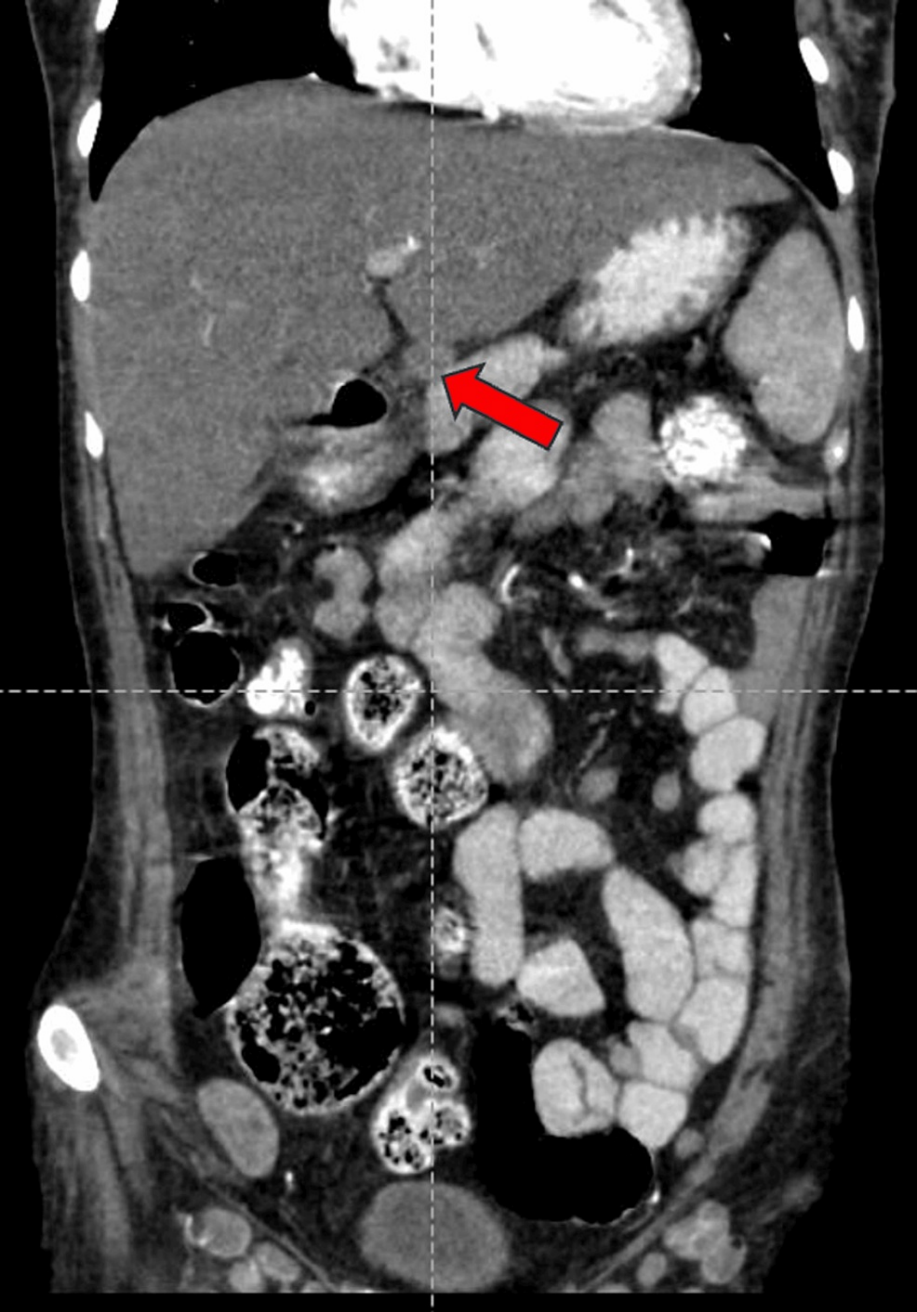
CECT-AP reformatted coronal section showing non-enhancing necrotic at the porta hepatis shown by the red arrow. CECT-AP: contrast-enhanced computed tomography of the abdomen and pelvis
